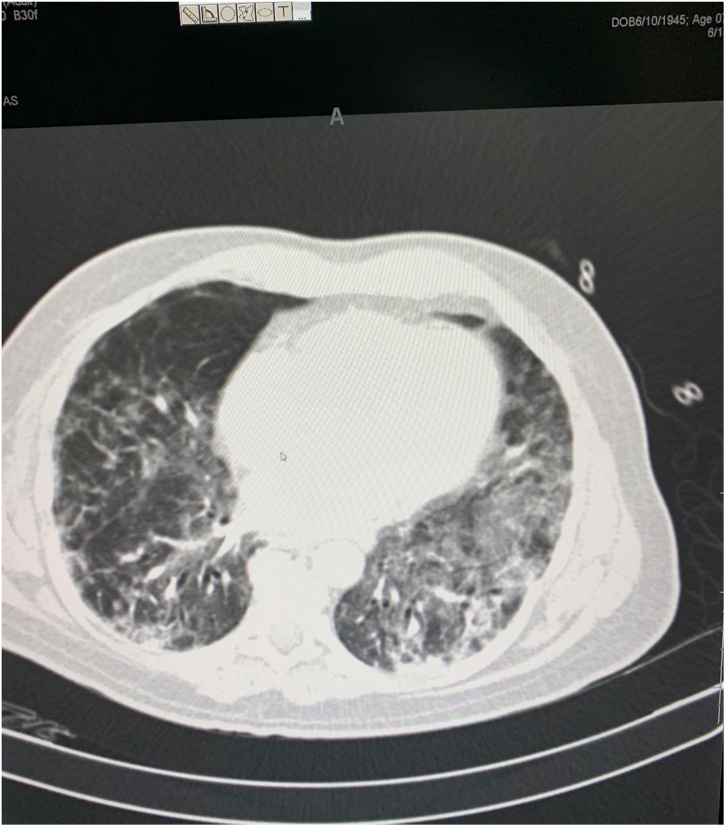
CT chest shows signs of bilateral COVID-19 pneumonia.
